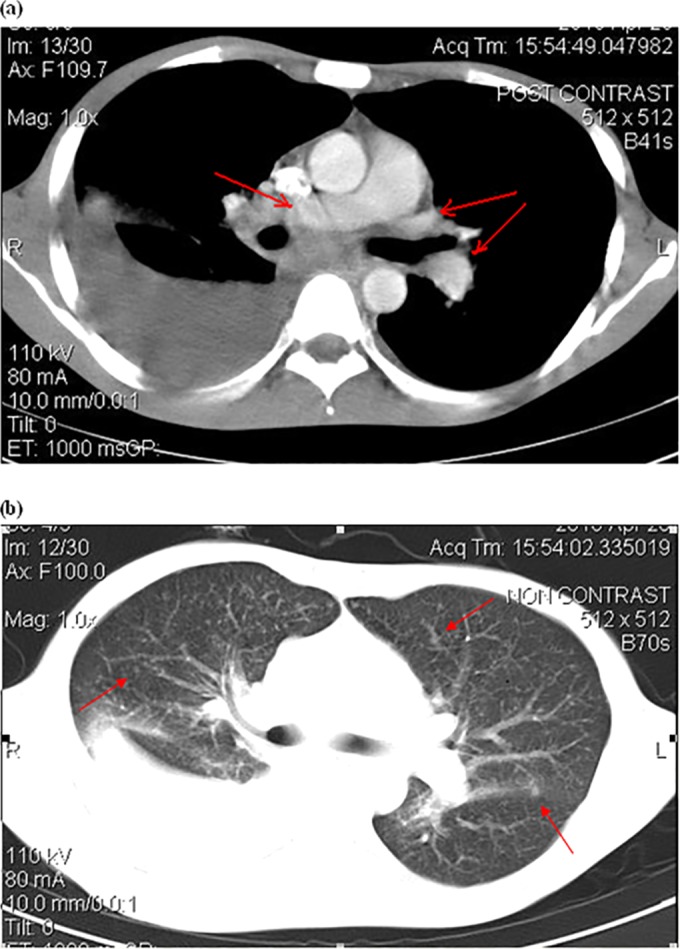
Chest computed tomography of patient 2 showing a large pleural effusion in the right lung. (a) Arrows indicate enlarged mediastinal lymph nodes; (b) arrows indicate parenchymal nodules.
